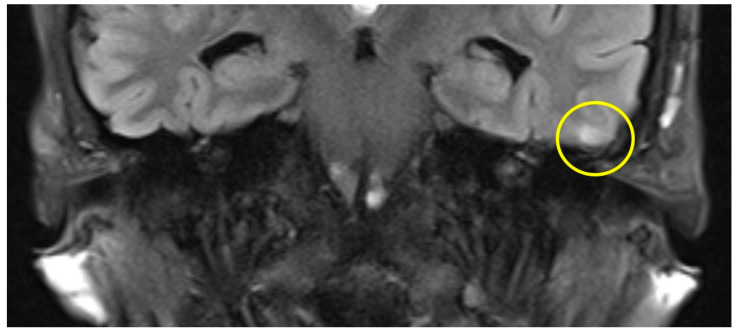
Coronal T2 flair MRI of patient #4 (yellow circle = gliosis). Supplementary MR imaging can be downloaded.
